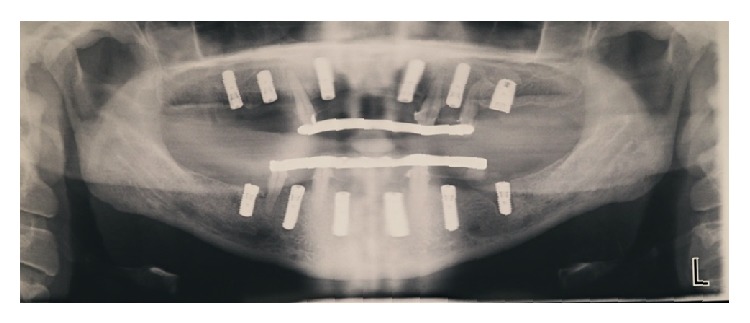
Panoramic radiograph shows the placement of six implants per arch. Note metal-reinforced fixed provisional prostheses retained by retained teeth.
